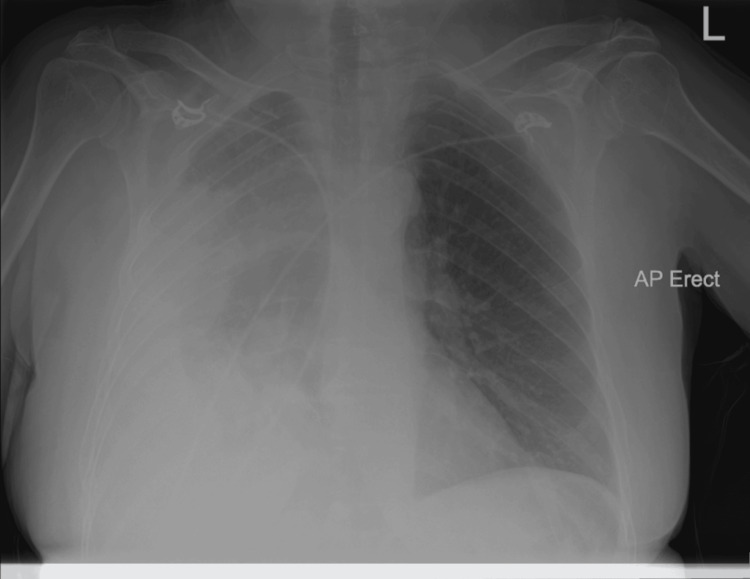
Chest X-ray performed at initial presentation showing a large right-sided pleural effusion.
